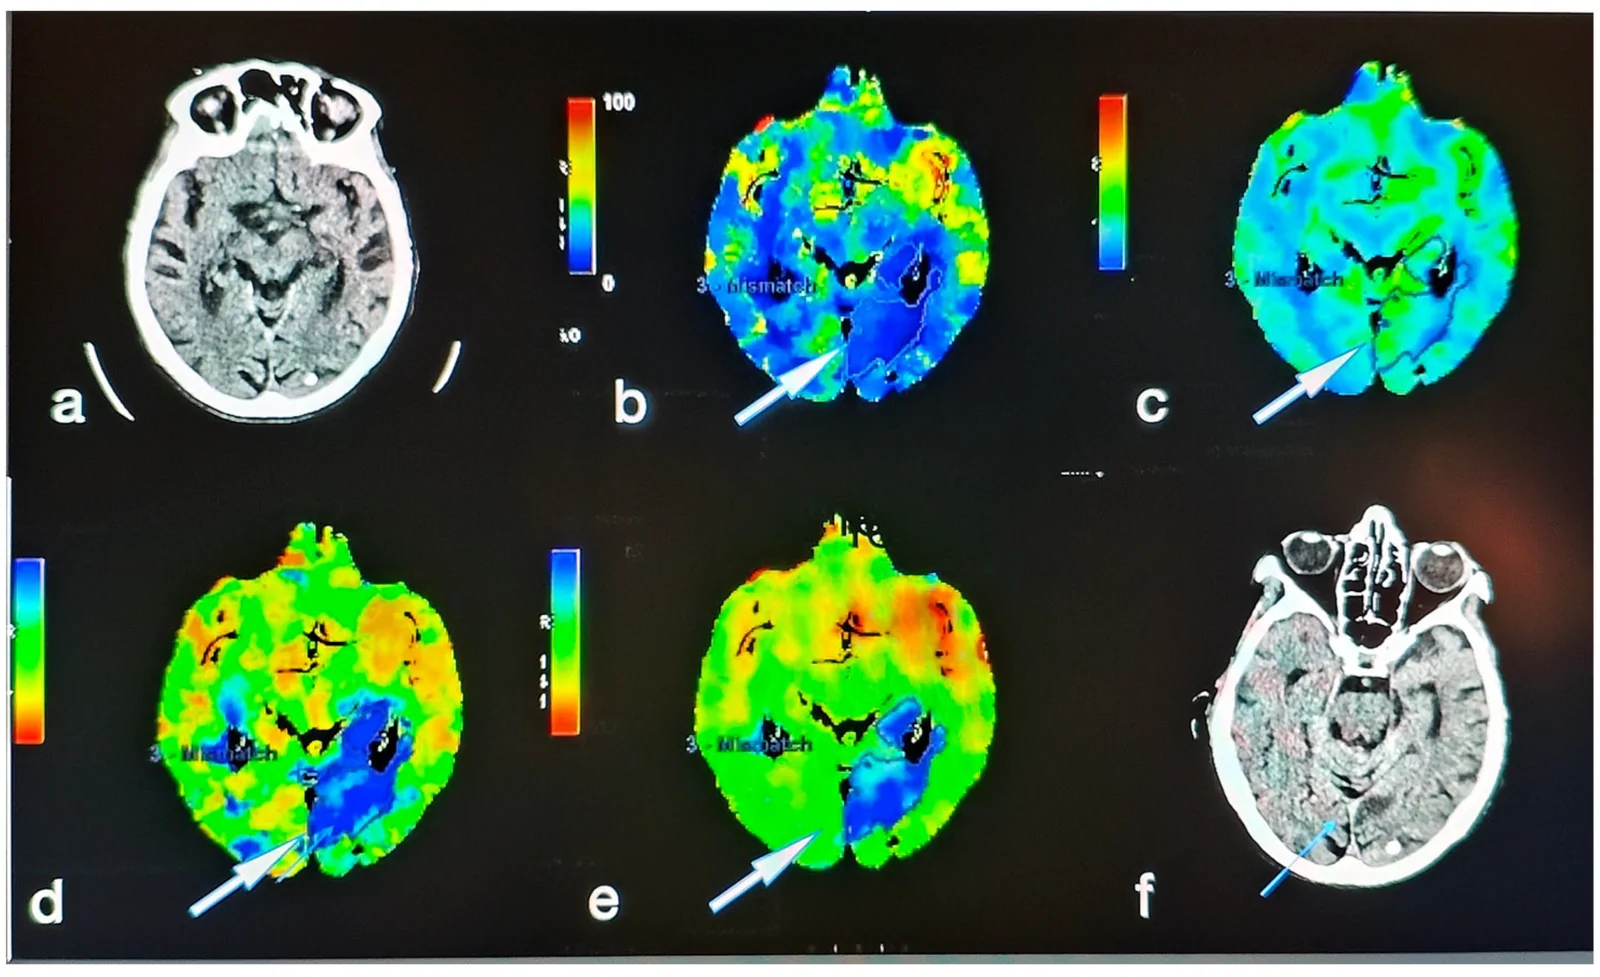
An 82-year-old female patient from Hospital “Saint Sava”, with instability and right-sided weakness. NCCT without pathological changes, ASPECT 10 (a). CTP showing a perfusion deficit in the ACP irrigation zone on the left at CBF (b) and CBV (c), with prolonged MTT (d) and Tmax (e) and a penumbra zone of 21.12 mL (white arrow). Follow-up NCCT after 24 h shows an acute ischemic lesion on the left occipital lobe (blue arrow) (f).
